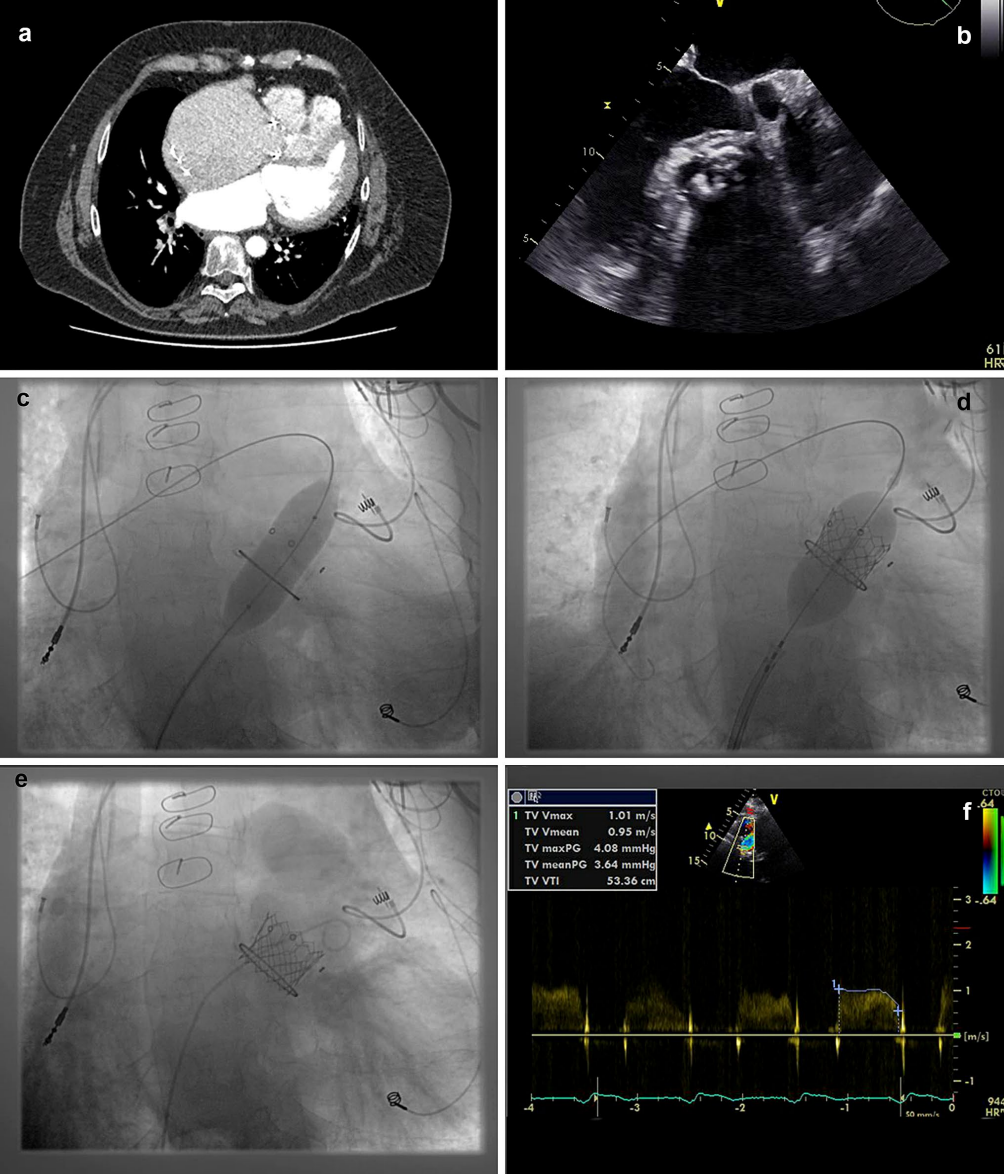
CT, transesophageal echocardiography, valve placement and final Doppler
